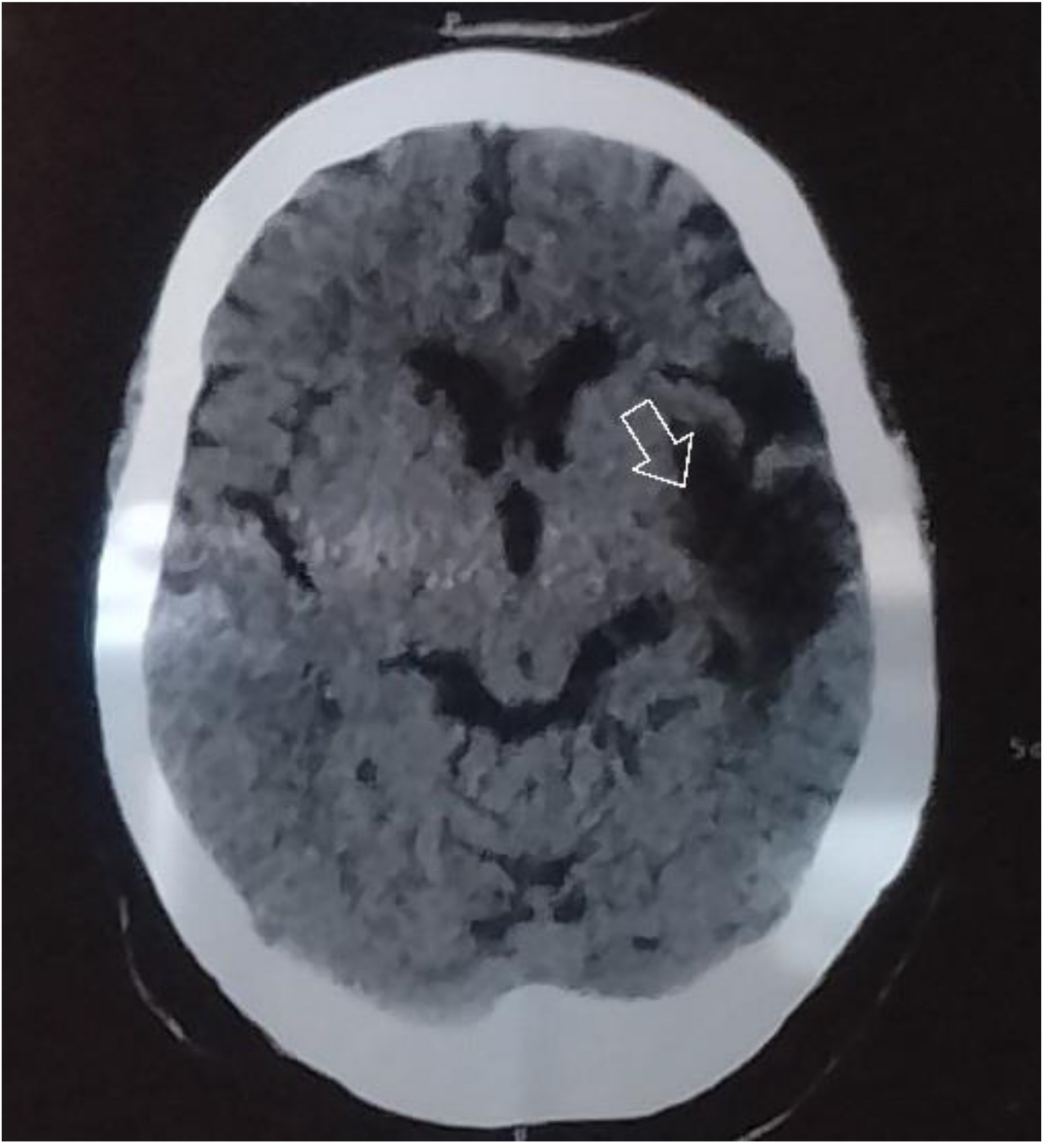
Head CT showing areas of infarction as described in the text.
